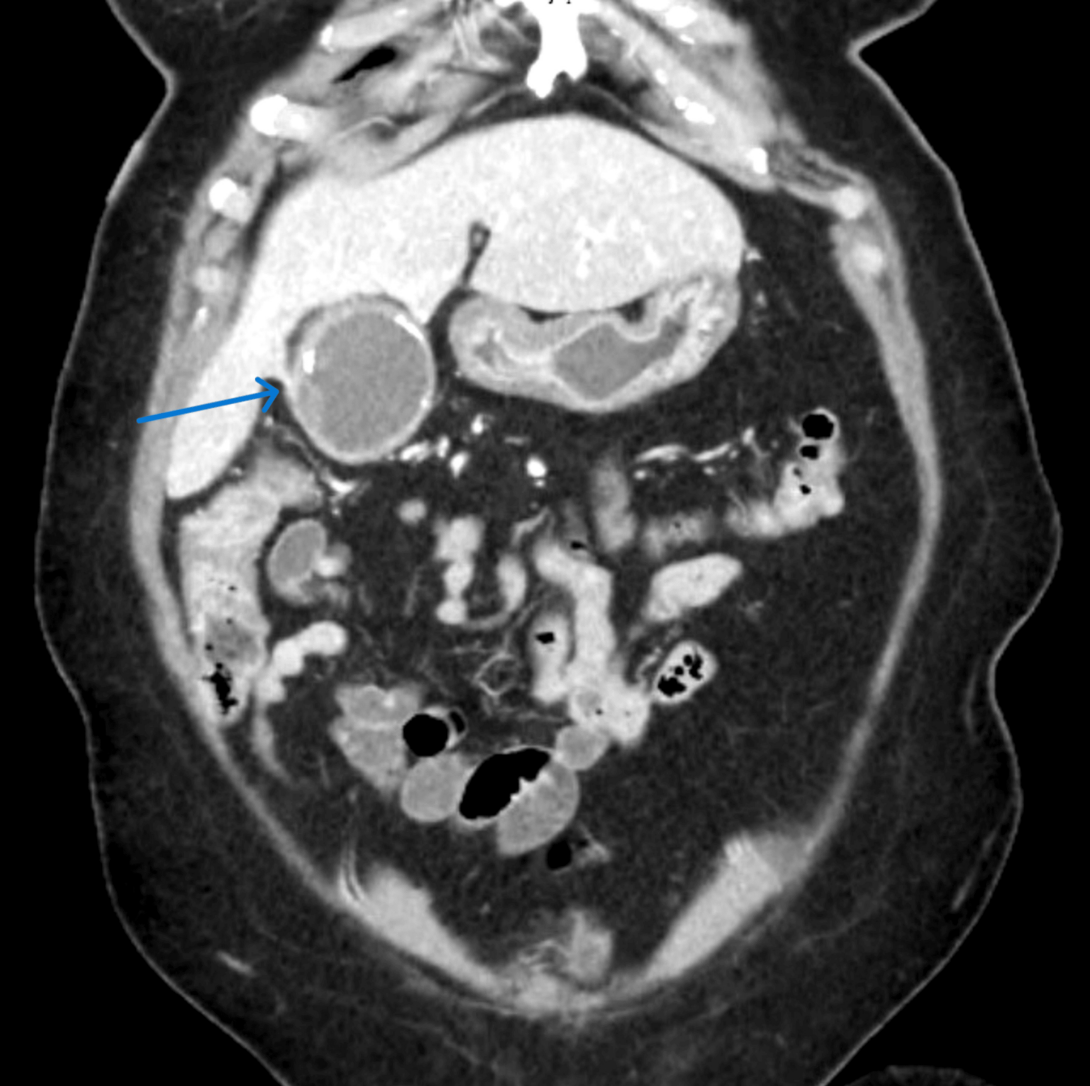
Preoperative computed tomography (CT) scan of the abdomen and pelvis demonstrating an inflamed gallbladder with gallbladder wall thickening and no evidence of metastatic disease.
